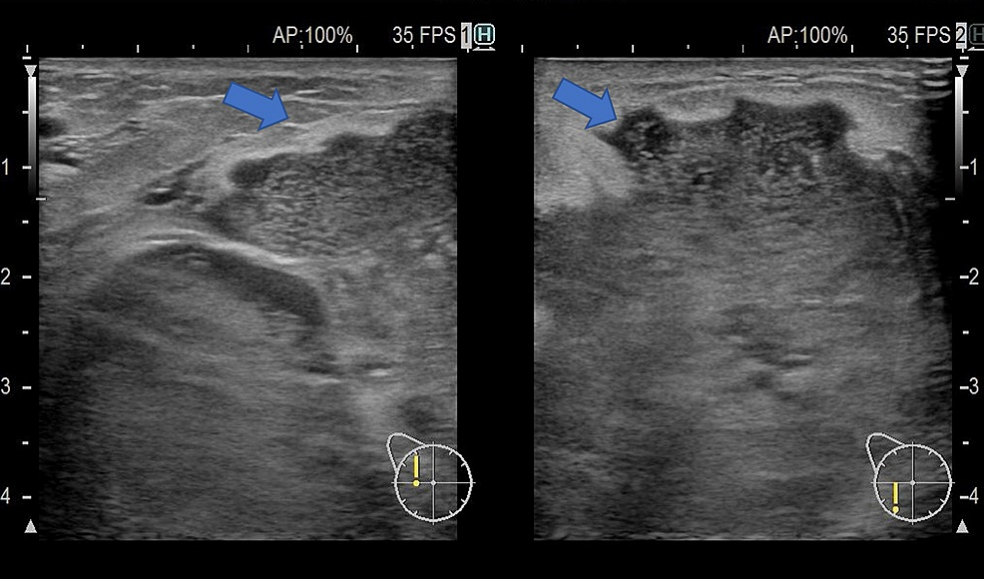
Ultrasonography of the right breast
